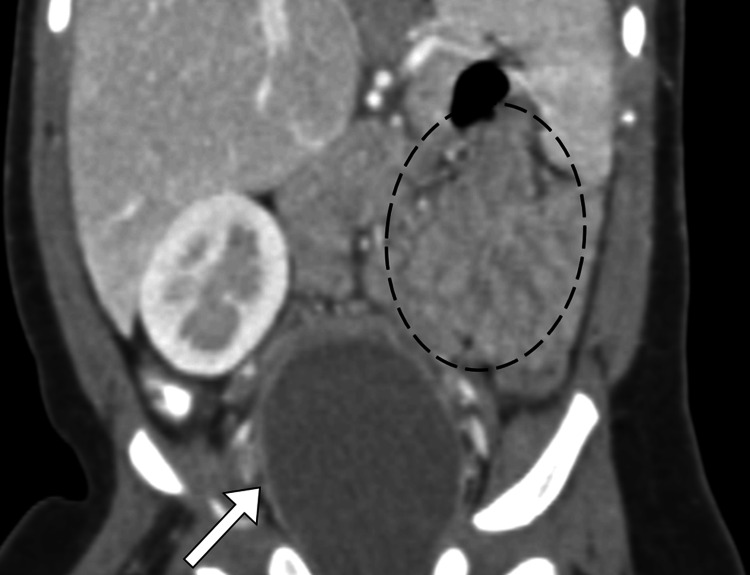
Coronal contrast-enhanced computed tomography image demonstrating a large retrovesical abdominopelvic cyst (arrow). The cyst is clearly visible in the pelvic region, with the additional finding of left renal agenesis (encircled).
